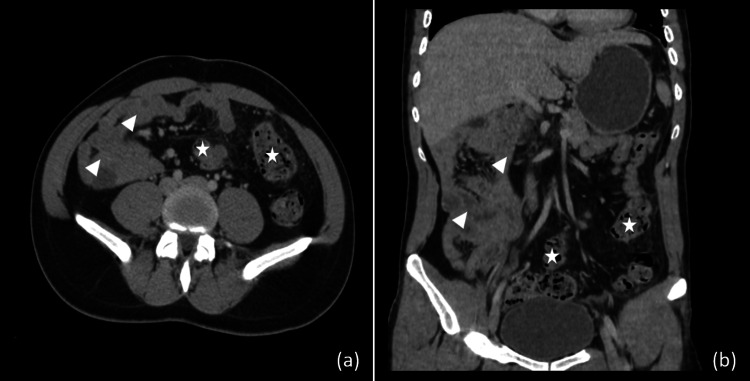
The CT scan shows, in axial (a) and coronal (b) sections, the colon on the left side of the abdomen (white star), and the small intestine on the right side (arrowhead).
